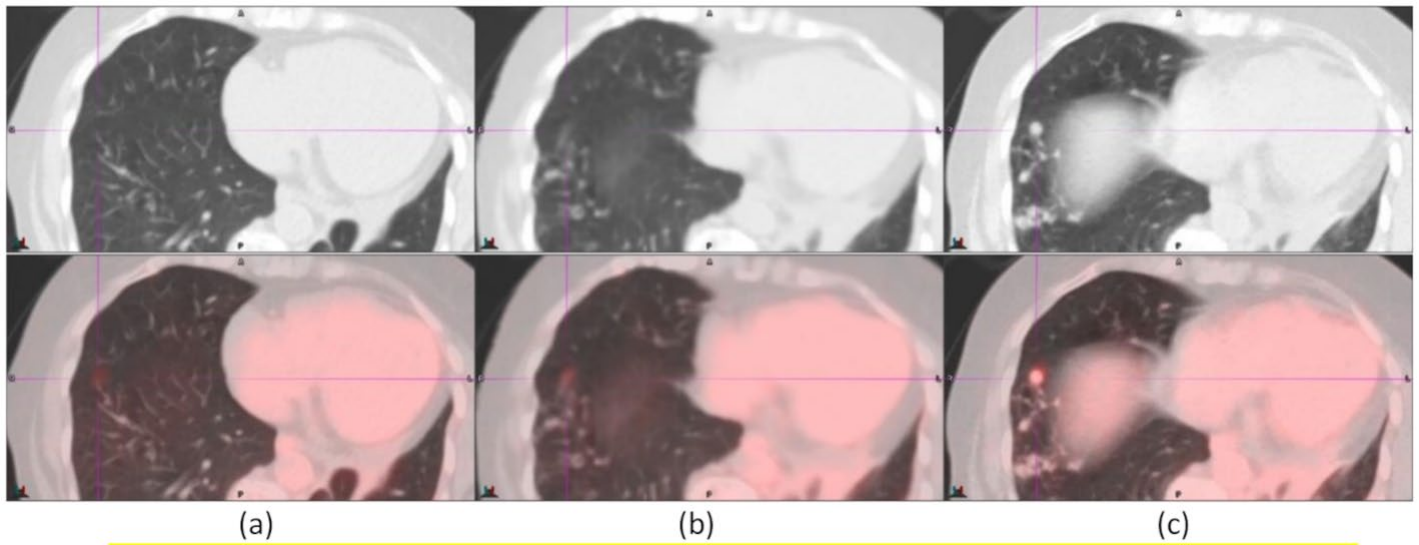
A 0.9-cm lung nodule was registered in DDG CT (c) but not in baseline (a) or AIR (b). The upper panel is CT, and the bottom panel is the fusion of PET and CT. The SUV_max_ values of the lung nodule were 1.87 for baseline, 2.08 for AIR and 4.21 for DDG CT
